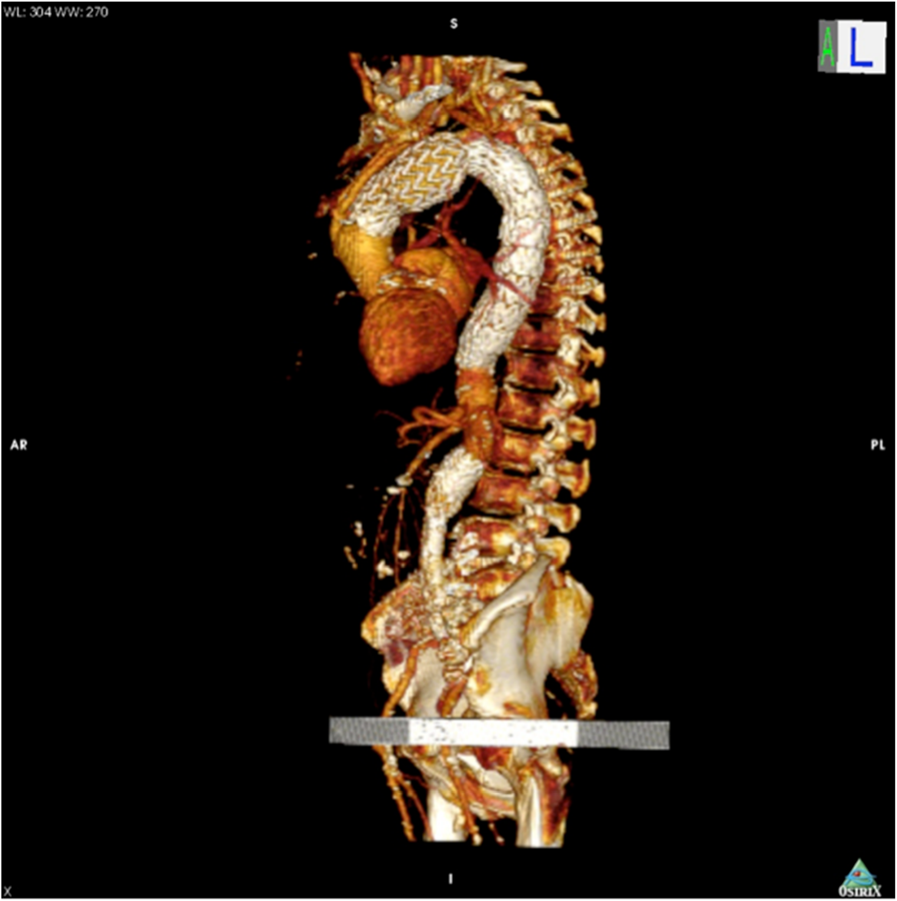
Sagittal CT angiogram three-dimensional (3D) reconstruction showing aortic arch and descending aortic stenting and aorto-bi-iliac prosthesis
